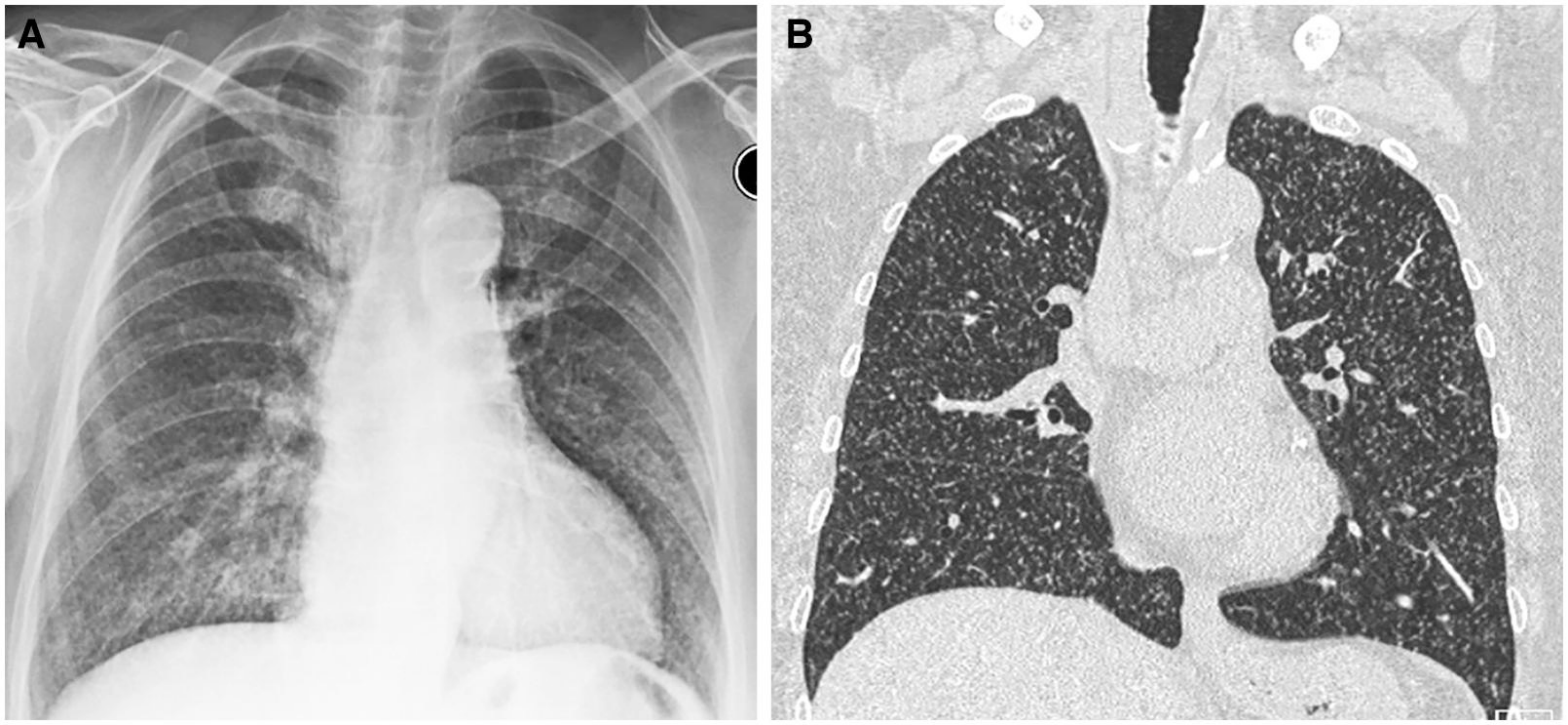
Miliary TB in a 67-year-old woman. (A) Frontal radiograph and (B) coronal CT image show numerous tiny miliary nodules in both lungs.
